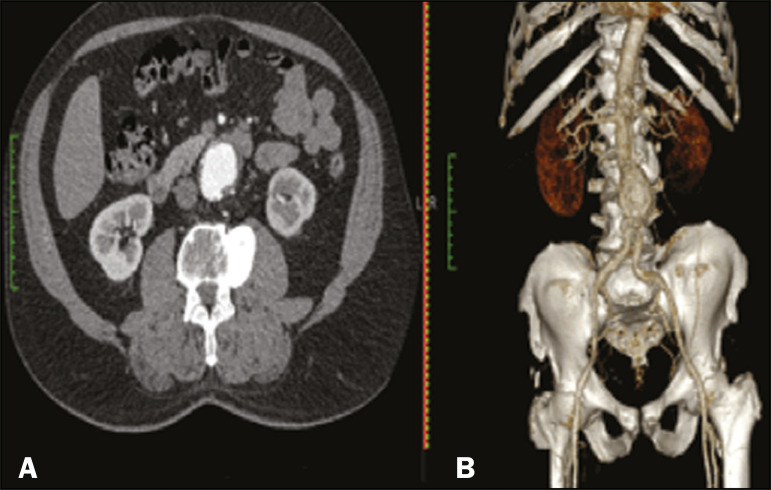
CTA of the abdominal aorta using the Horos software, in axial and 3D views (A and B, respectively).
